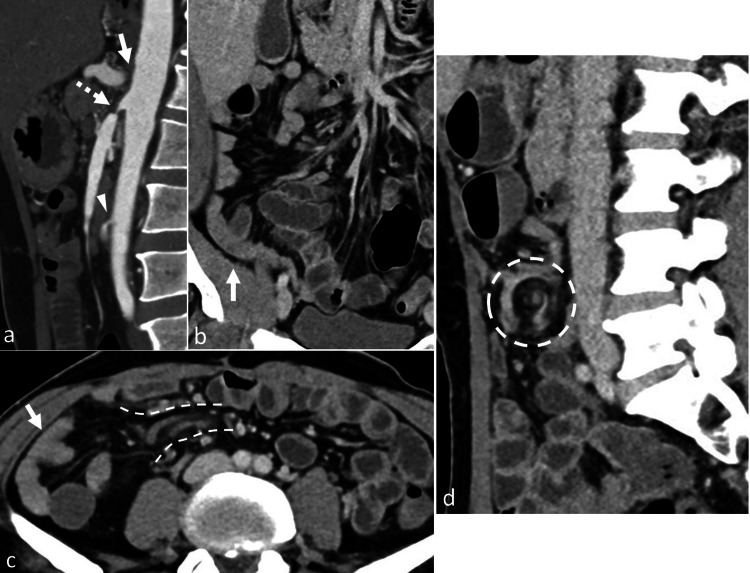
Chronic mesenteric ischemia with transmesenteric hernia (a) Sagittal reformatted arterial phase CT image showing the short-segment occlusion of the celiac axis ostium (arrow) and short-segment high-grade stenosis of the ostia of the superior (dashed arrow) and inferior (arrowhead) mesenteric arteries. (b, c) Coronal reformatted (b) and axial (c) venous phase CT images showing the clustering of ileal loops in the right iliac fossa (arrows), with a perpendicular orientation of its vascular pedicle (outlined by dashed lines in c) to the other branches of the superior mesenteric artery, suggestive of transmesenteric hernia. (d) Sagittal reformatted venous phase CT image showing the swirling of the vascular pedicle (outlined by dashed circle) of the hernia sac CT: computed tomography
